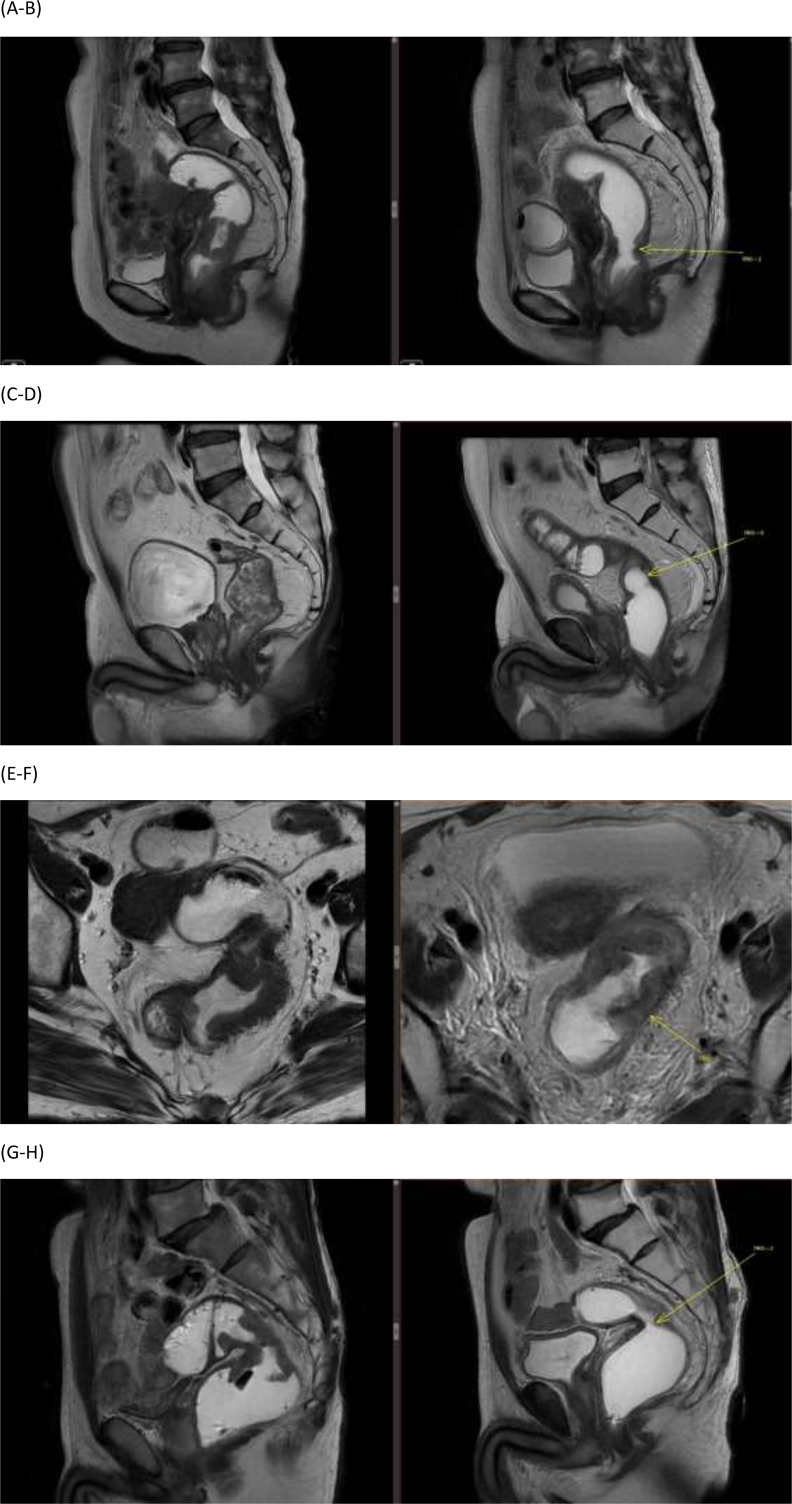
MRI assessment of tumor response before total neoadjuvant therapy (TNT) and at preoperative restaging after TNT (prior to surgery). (A, B) Sagittal T2-weighted MRI before TNT and at preoperative restaging after TNT showing partial response, confirmed by pathologic TRG = 2. (C, D) Sagittal T2-weighted MRI before TNT and at preoperative restaging after TNT showing complete response, confirmed by pathologic TRG = 0 (E, F) Axial T2-weighted MRI before TNT and at preoperative restaging after TNT showing partial response (PR), confirmed by pathologic TRG = 2. (G, H) Sagittal T2-weighted MRI before TNT and at preoperative restaging after TNT showing partial response, confirmed by pathologic TRG = 2.
